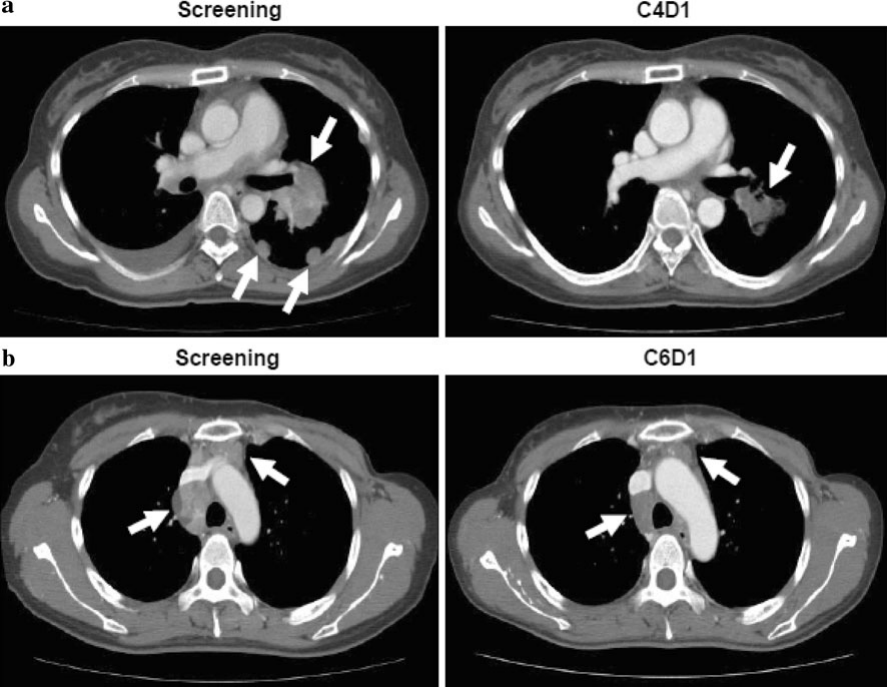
CT images for two patients with confirmed partial responses following linifanib treatment. a A 39-year-old female patient with lung cancer who received prior chemotherapy had lesions in the lung, pleura, and lymph nodes. This patient had a confirmed PR in C4, following linifanib treatment at 0.25 mg/kg. *Arrows* indicate tumor location at screening and at C5D1. b A 42-year-old female patient with breast cancer who received prior chemotherapy had target lesions in the mediastinal lymph nodes. This patient had a confirmed PR in C2, following linifanib treatment at 0.20 mg/kg. *Arrows* indicate tumor location at screening and at C6D21. Abbreviations: *CT* computerized tomography; *PR* partial response; *C* cycle; *D* day
